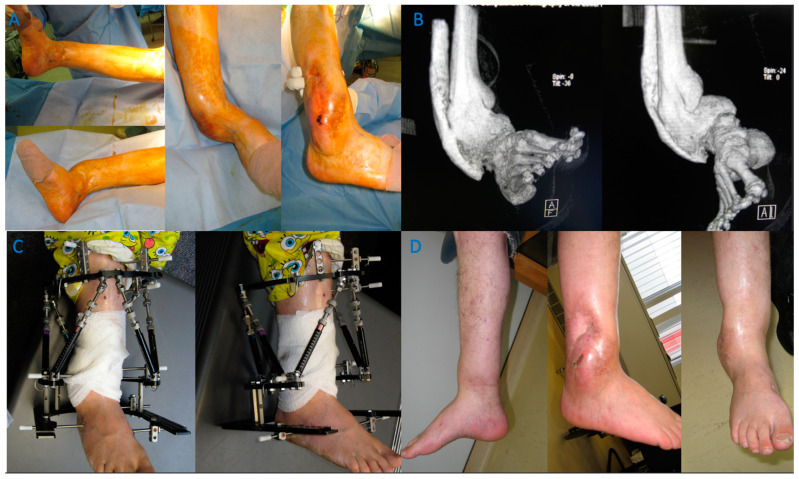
A case of a severe distal tibia deformity with obvious soft tissue damage on the lateral border of the deformity. Conventional X-rays were unable to fully assess or define the deformity that can be seen on a 3D reconstruction CT scan (B). Image (A) depicts a gross image. An osteotomy and fixation with a Tayler Spatial Frame (C) were used with a residual calculation to slowly correct the deformity. Slow correction would help protect the soft tissue envelope on the lateral border and stretch the medial soft tissues. Correction has resulted in a plantigrade foot with motion of the ankle and a healed tibia (D).
